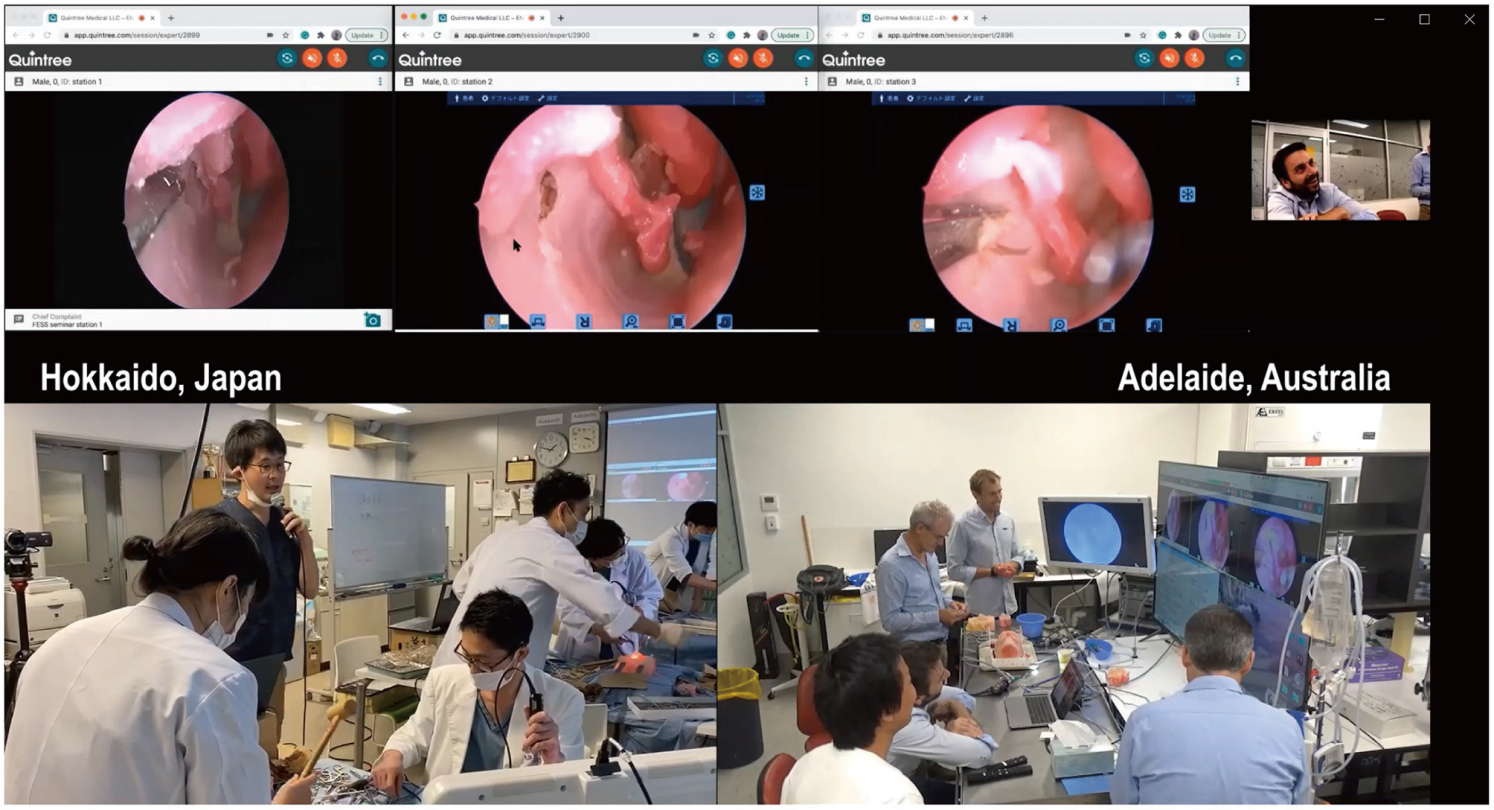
The remote endoscopic surgery training course was conducted between Hokkaido, Japan, and Adelaide, Australia, and broadcasted worldwide. The course was held over 2 days in February 2021. Three otolaryngologists in Hokkaido were trained to perform frontal sinus dissections on 3D sinus models printed from CT scans of patients. Two rhinologists based in Adelaide, 8,000-km away from Hokkaido, simultaneously viewed the surgeries and provided real-time feedback using a telemedicine platform, Quintree. The course was also broadcast worldwide to over 200 otolaryngologists' personal computers.
